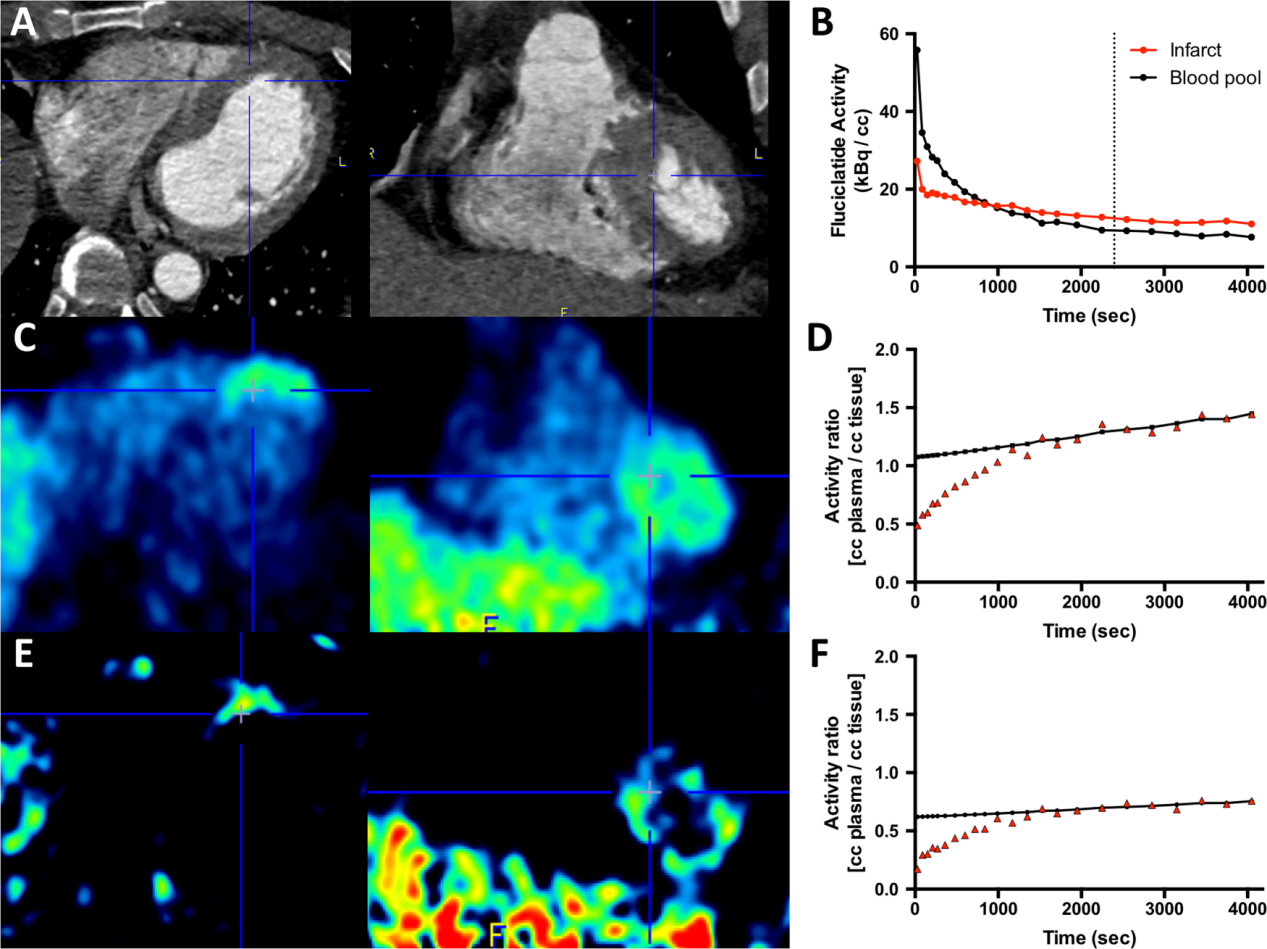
Dynamic analysis of ^18^F-fluciclatide uptake is shown. Axial and sagittal CT angiographies of the thorax in a patient with recent anterior myocardial infarction (A) are shown. The time-activity curves generated from the descending aorta and the apical interventricular septum (blue crosshairs) show increased uptake in the infarct relative to blood pool. Optimal contrast between ^18^F-fluciclatide tissue and blood pool activity was observed after 40 min (dotted line, B). The positron emission tomography image in the axial and sagittal plane shows increased uptake within the apical septum, although there is some background activity (C). Patlak analysis of regions of interests placed in the interventricular septum confirms integrin binding, as evidenced by the gradient of the slope and the y-intercept (D) and, using a *K*i-generated image, we can better identify and delineate focal uptake within myocardium (E). A region of remote myocardium within the same patient generates a Patlak curve with significantly lower gradient and intercept in comparison (F).
